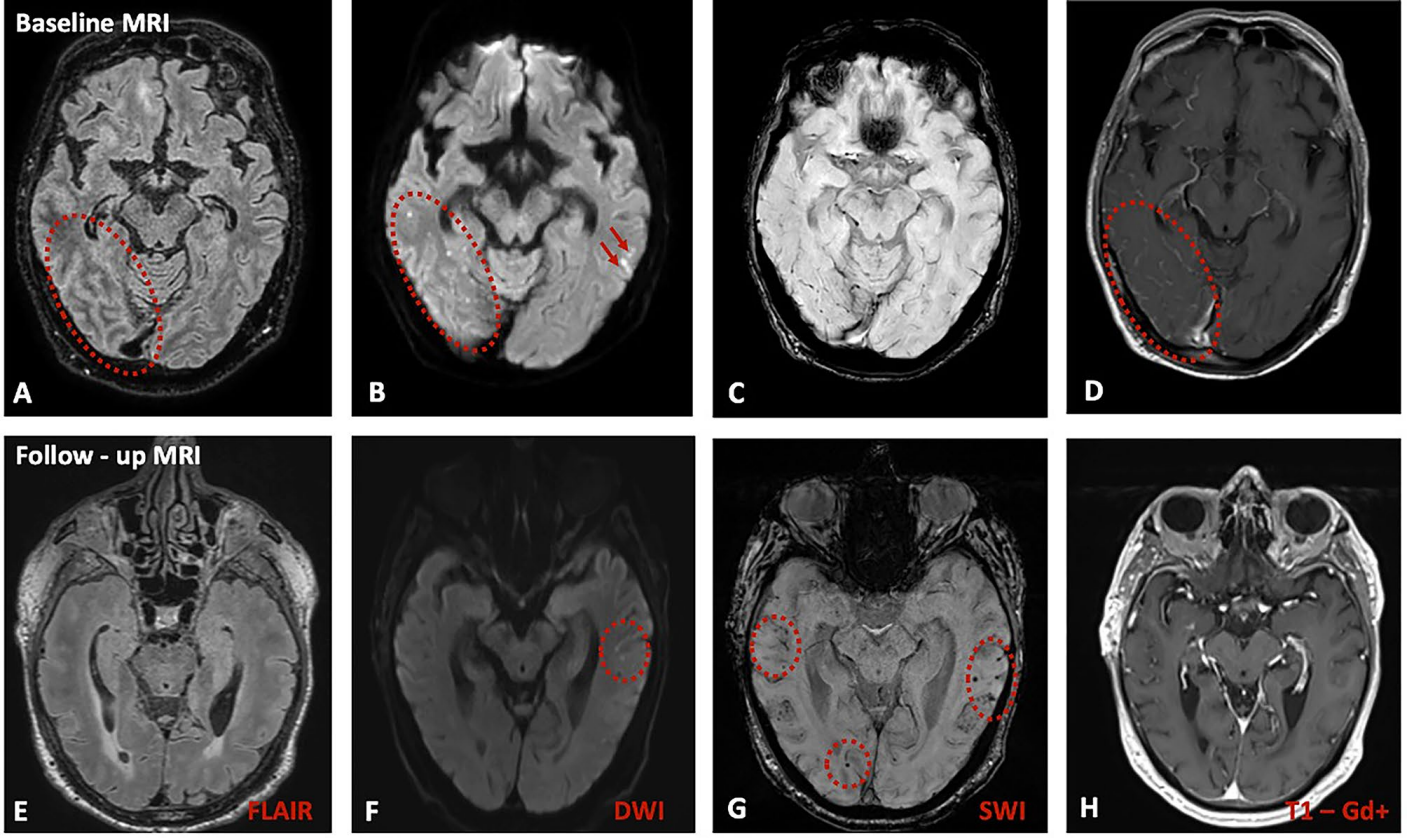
MRI findings at initial presentation and after corticosteroid treatment. Baseline-MRI showed FLAIR subarachnoid hyperintensities (Panel-A; red dotted circle), multiple cortical/subcortical microinfarcts in Diffusion-Weighted-Imaging (Panel-B; red dotted circle and red arrows), scarce microbleeds in Susceptibility-Weighted-Imaging (Panel-C) and leptomeningeal gadolinium-enhancement right parietooccipital (Panel-D; red dotted circle). Follow-up Imaging two months post-corticosteroid initiation disclosed resolution of FLAIR-hyperintense lesions (Panel-E), new subcortical microinfarcts (Panel-F; red dotted circle), increase of cerebral microbleeds (Panel-G; red dotted circles) and complete resolution of gadolinium-enhancement (Panel-H)
